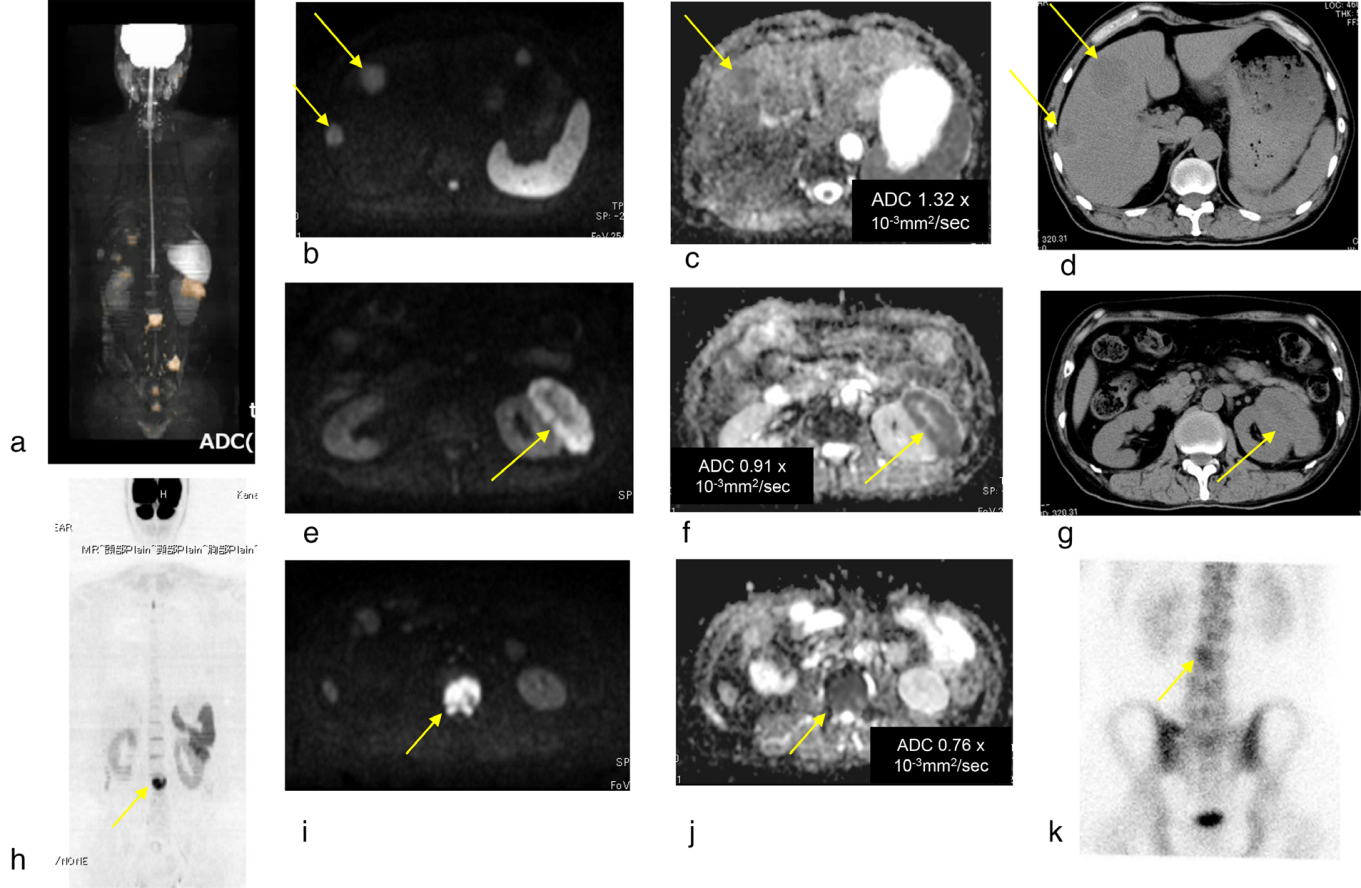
Case 1. Large cell neuroendocrine carcinoma (LCNEC) pT2aN0M0. (a) Whole body imaging of diffusion‐weighted whole‐body imaging with background suppression (DWIBS) show metastases in the liver, kidney, vertebra and ilium. (b) Multiple liver metastases were present on DWIBS, (c) apparent diffusion coefficient (ADC) map, and (d) CT. (c) The ADC of a liver metastasis was 1.32 × 10^−3^ mm^2^/s. Left renal metastasis was present on (e) DWIBS, (f) ADC map, and (g) CT. The ADC of the left renal metastasis was 0.91 × 10^−3^ mm^2^/s (f). (h, i) Metastases to lumbar vertebra is shown in DWIBS and (k) in bone scintigraphy. (j) The ADC of the one lumbar vertebra metastasis was 0.76 × 10^−3^ mm^2^/s. DWIBS showed the lumbar vertebra metastasis more clearly than bone scintigraphy
